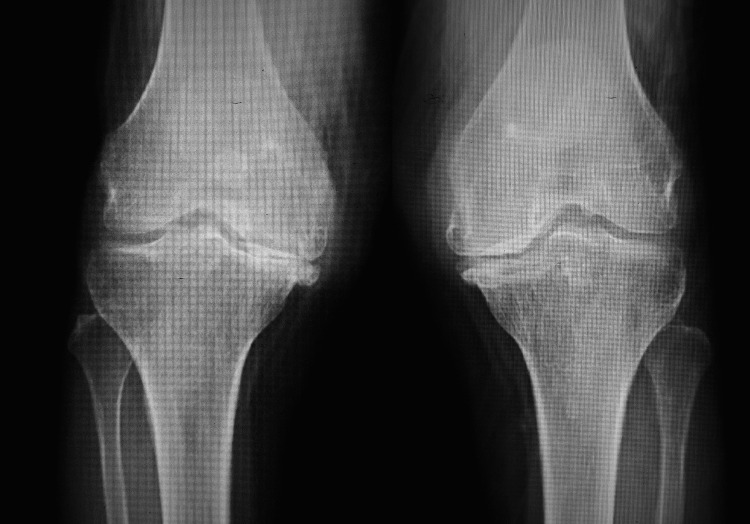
Anteroposterior radiograph of the bilateral knee in standing posture with grade IV osteoarthritis with large osteophytes, narrowing of joint spaces, marked sclerosis of the medial joint, and varus deformity of both knees.
